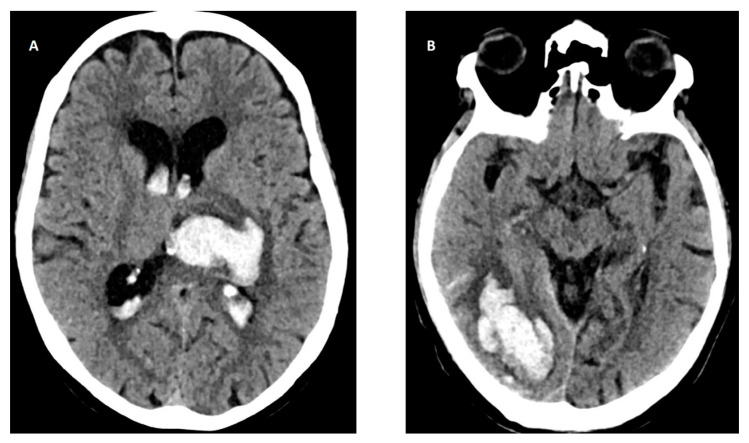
Head CT of intracerebral hemorrhage (ICH). (A) Deep basal ganglia ICH in the left hemisphere with intraventricular hemorrhage (IVH) due to hypertension. (B) Lobar ICH in right occipital lobe with subarachnoid hemorrhage (SAH) probably due to cerebral amyloid angiopathy (CAA).
